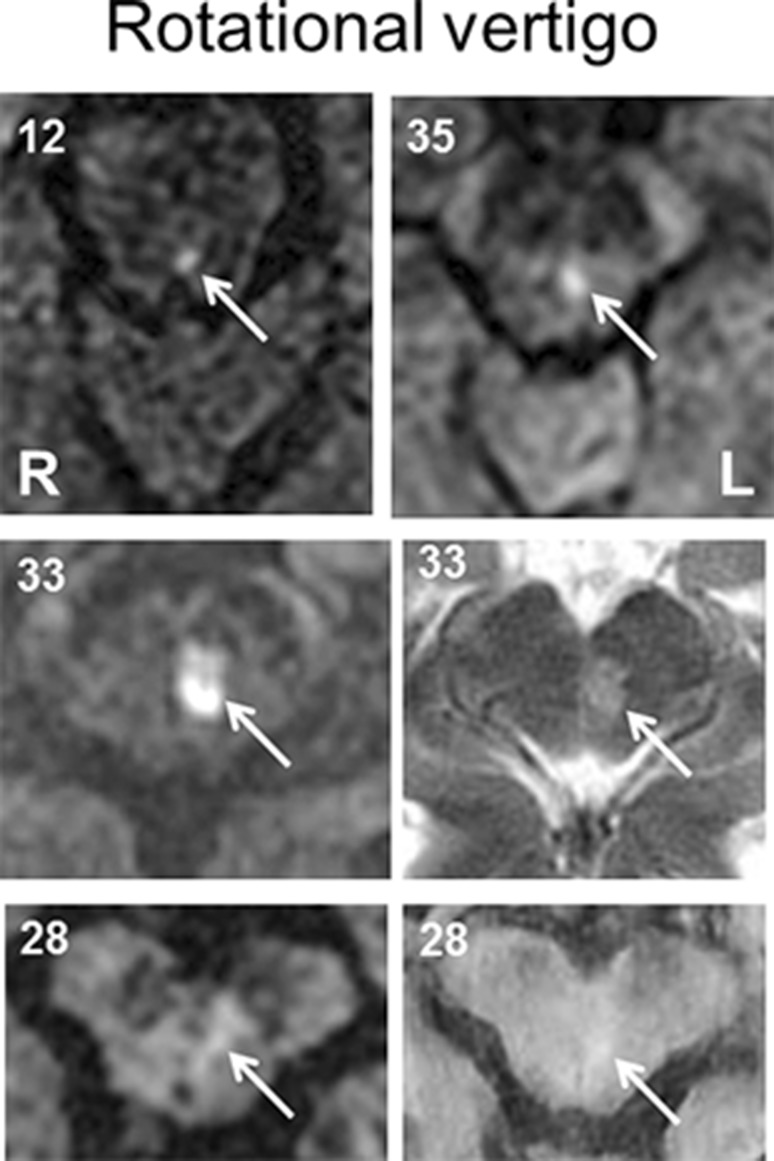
MRI scans of four patients (nos. 12, 28, 33, and 35) who manifested with acute transient rotational vertigo due to circumscribed unilateral midbrain strokes (diffusion- and T2-weighted sequences). In the upper panel two transversal sections of the caudal midbrain (no. 35; with left internuclear ophthalmoplegia, INO) and the midbrain close to the pons (no. 12, with left INO) are depicted with small unilateral infarctions of the left tegmentum affecting the medial longitudinal fascicle which contains ascending vestibular fibers. In the other two patients (no. 33 with vertical gaze palsy and convergence retraction nystagmus, and no. 28 with vertical gaze palsy and fascicular fourth nerve palsy right) the unilateral strokes involve a midbrain level close to the oculomotor nucleus, the interstitial nucleus of Cajal, and the rostral interstitial nucleus of the MLF, see also Table 1
